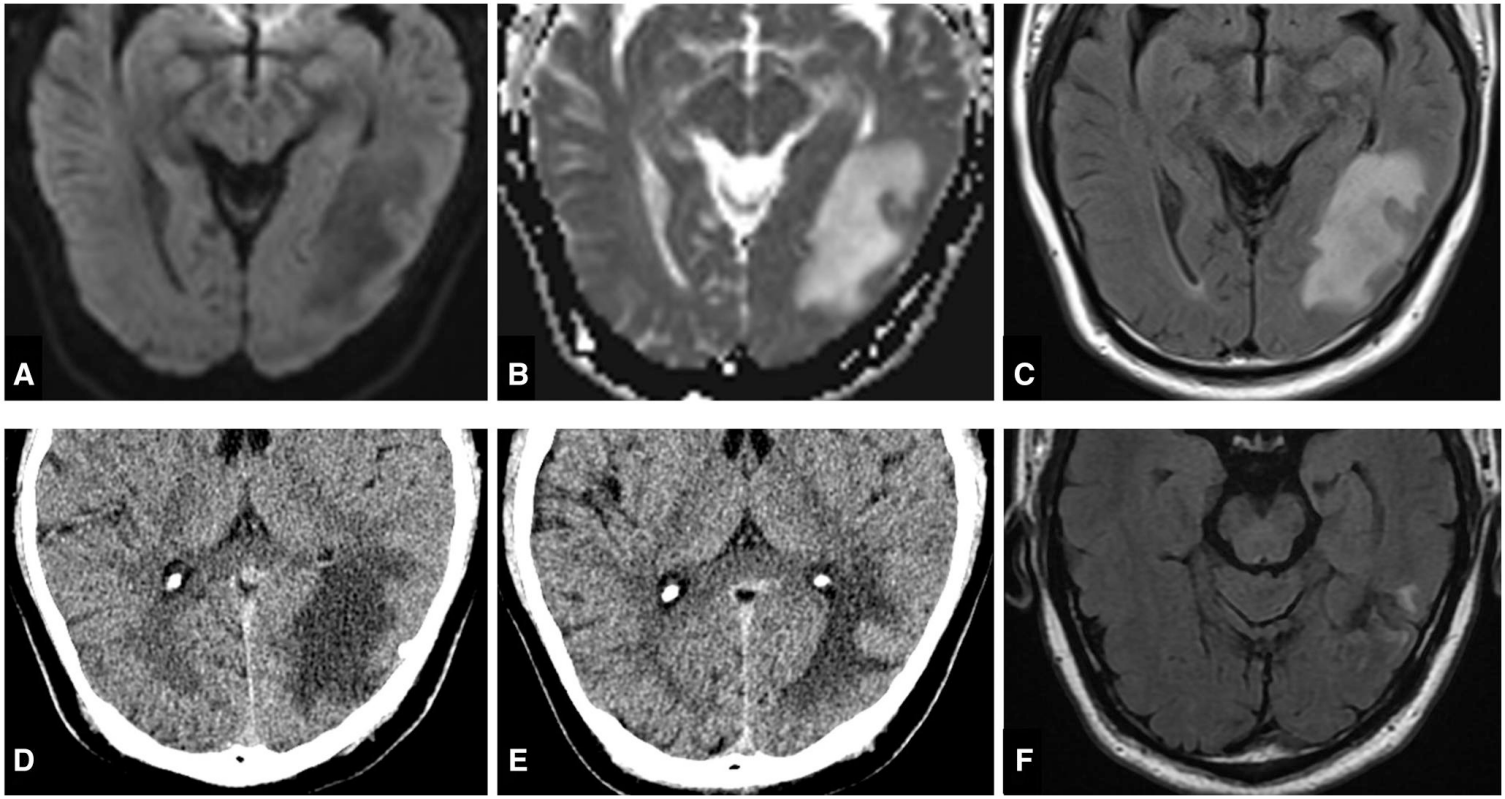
(A) Diffusion weighted imaging (DWI), (B) Apparent diffusion coefficient (ADC), and (C) T2-fluid-attenuated inversion recovery (T2-FLAIR) images 3 days after embolization revealed regional low DWI signal and high ADC value and T2FLAIR signal at left temporooccipital white matter. (D) CT images displayed white matter low densities at the same region. After treatment with enoxaparin and dexamethasone, (E) follow-up CT images in 2 weeks demonstrates gradually decreasing white matter oedema. (F) Follow-up T2-FLAIR images in 3 months showed minimal residual gliosis.
